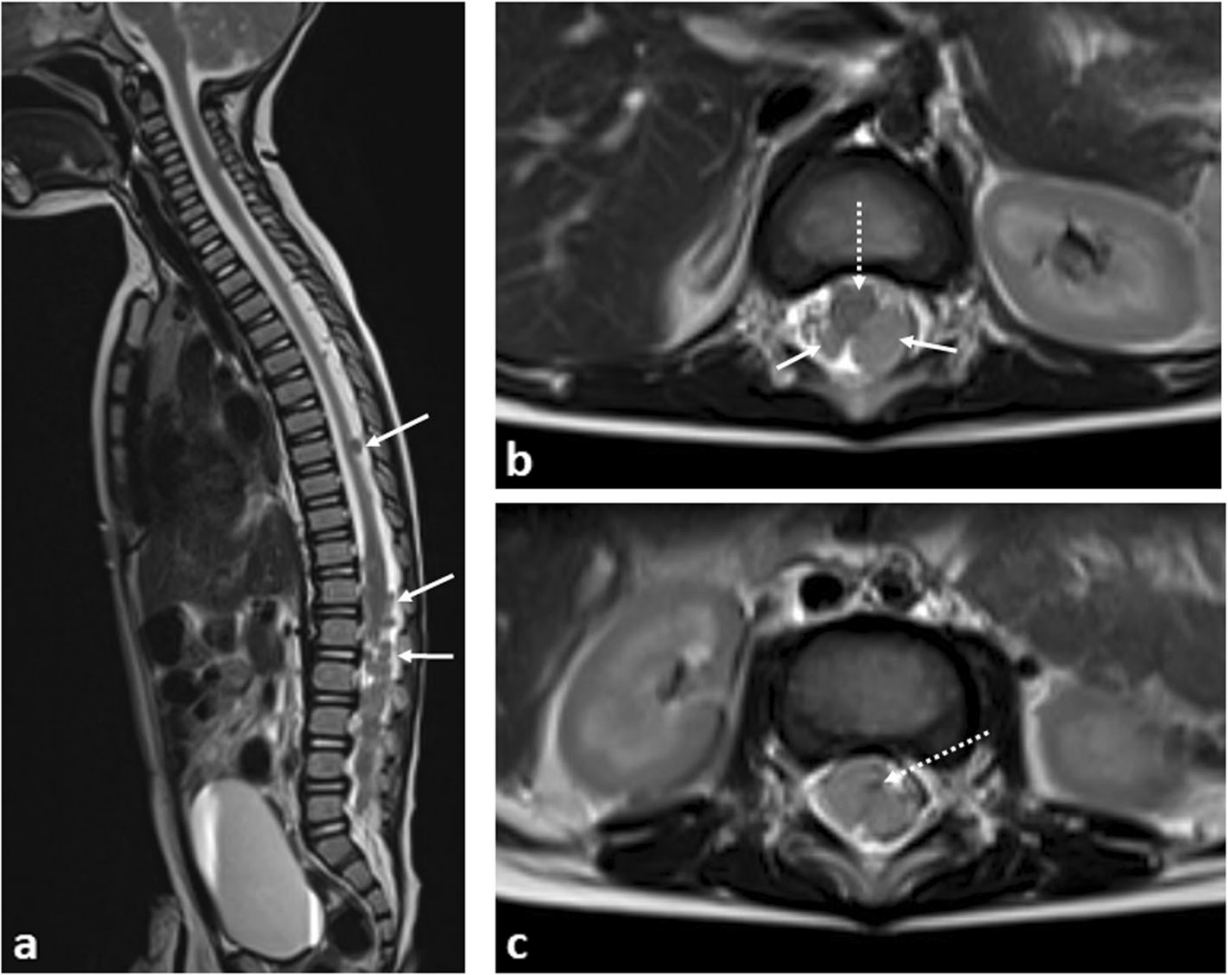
A 9-month-old boy with disseminated spinal leptomeningeal disease secondary to an intracranial ATRT. a Sagittal T2-weighted MRI of the spine demonstrates numerous extra-medullary, intraspinal tumour deposits (arrows). b Axial T2-weighted MRI of the lumbar spine demonstrate how these deposits (arrows) occupy the majority of the spinal canal, with the spinal cord (dashed arrow) anteriorly displaced, and (c) eventually becoming compressed at the cauda equina, where it is barely visible (dashed arrow). Emergency intrathecal chemotherapy and radiotherapy was subsequently performed
